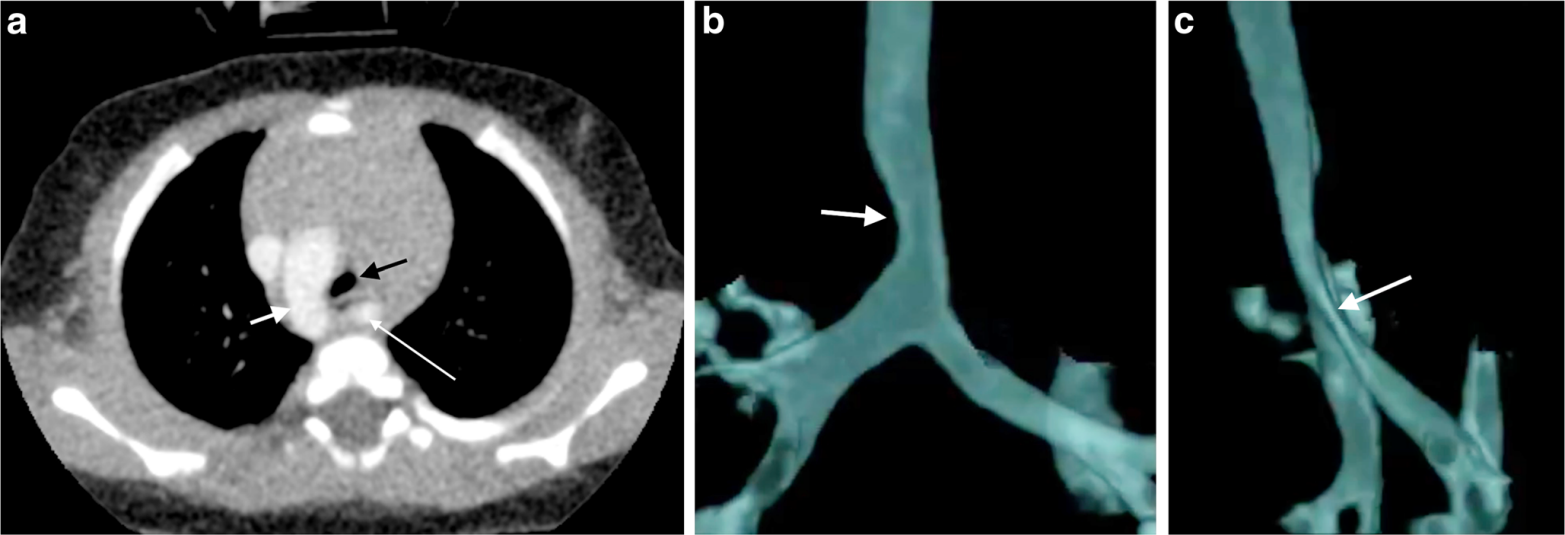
Dynamic 4-D CT study of the airways in a 4-month-old boy with a right aortic arch and aberrant left subclavian artery (the dynamic representation of this imaging is Supplementary material 5). a Axial slice of one of the four post-contrast CT scan phases of the dynamic 4-D CT scan demonstrates the right aortic arch (*short white arrow*) and course of the aberrant left subclavian artery (*long white arrow*) in the traditional axial plane. The tracheal compression (*black arrow*) from the right anteriorly by the aorta and posteriorly by the aberrant left subclavian artery is demonstrated. b Anterior perspective of a 3-D volume-rendered reconstruction from a dynamic 4-D CT demonstrates a fixed impression on the right side of the trachea (due to the right-side aortic arch) (*white arrow*) bronchial sites of stenosis. c Lateral perspective of a 3-D volume-rendered reconstruction from a dynamic 4-D CT demonstrates the severity of the tracheal stenosis best, where the trachea and left main bronchus are compressed from posterior by the aberrant left subclavian artery (*white arrow*)
